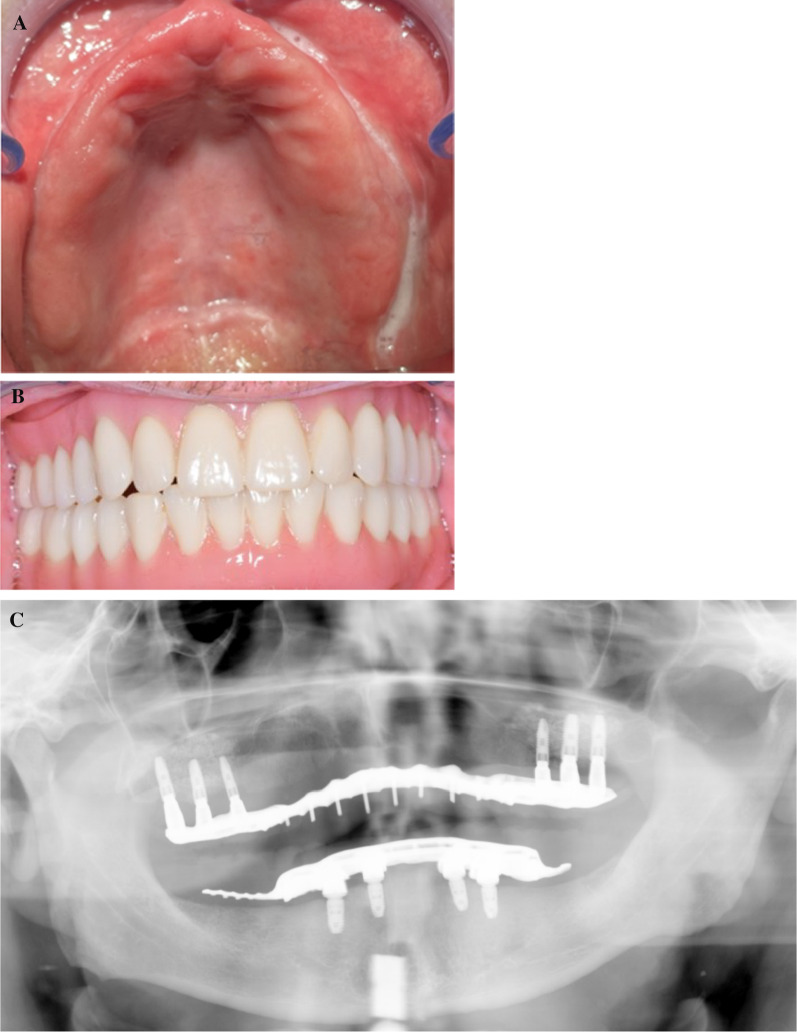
Final overview of restorative dental care. A Palatal view of the bar prosthesis in the maxilla. B Frontal view of the final situation. C Panoramic radiograph showing good integration of the augmentation material and the implants
